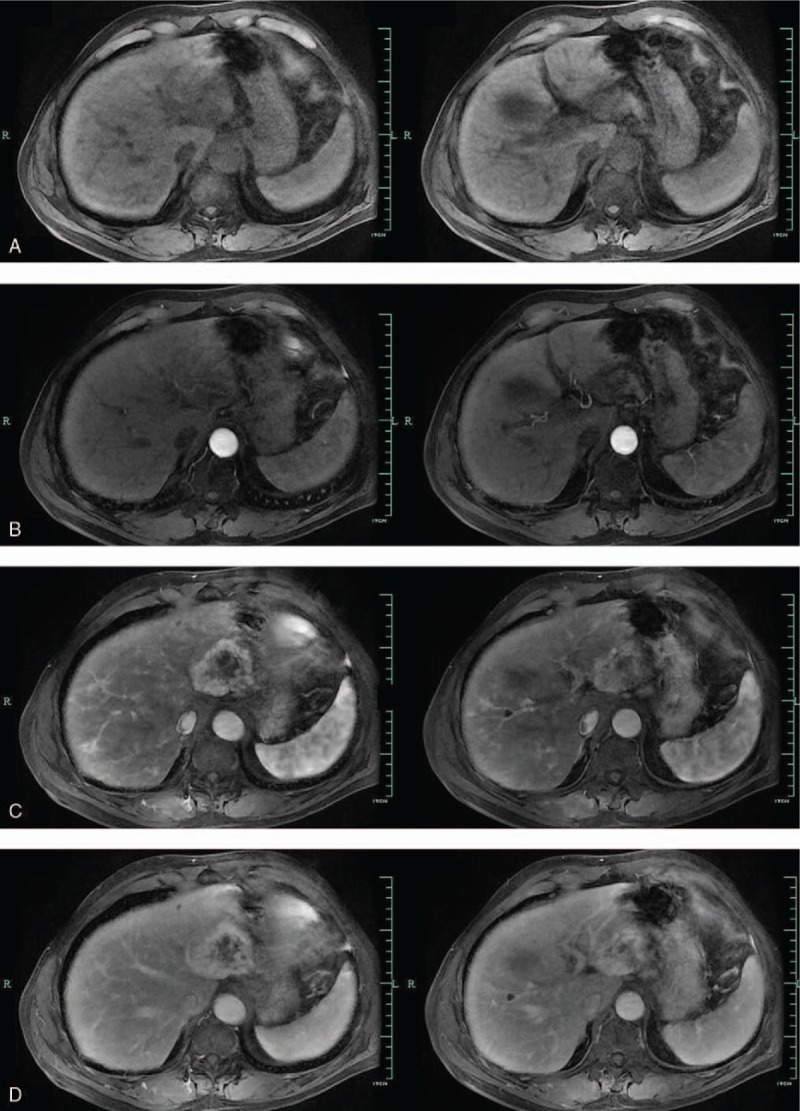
Dynamic contrast-enhanced Magnetic resonance imaging (MRI) scans. (A) Precontrast MRI scan shows a large, heterogeneous mass with low signal intensity in liver. (B) Arterial phase of dynamic, contrast-enhanced MRI scan showed no obvious uptake. (C and D) Portal phase and delayed phase of MRI scans showing gradual progressive centripetal enhancement from peripheral to central areas, except for central areas of low signal intensity.
